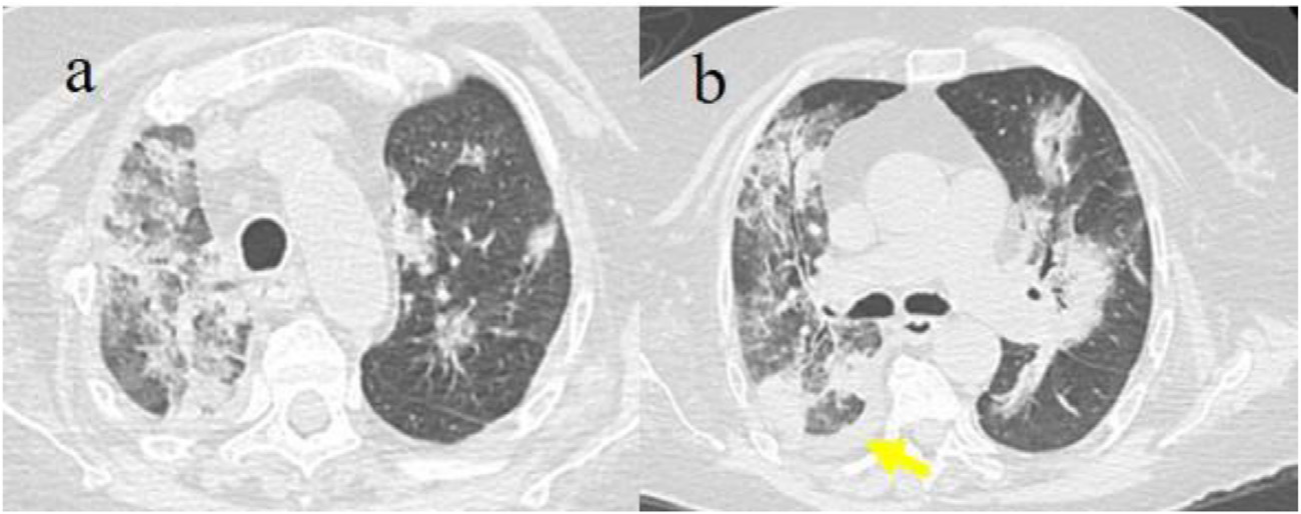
Chest-CT performed 1 week later. In the image “a” and “b” is represented a more pronounced consolidations of the COVID-19 pneumonia in the superior lobes with a mild pleural effusion on the right side (yellow arrow).
